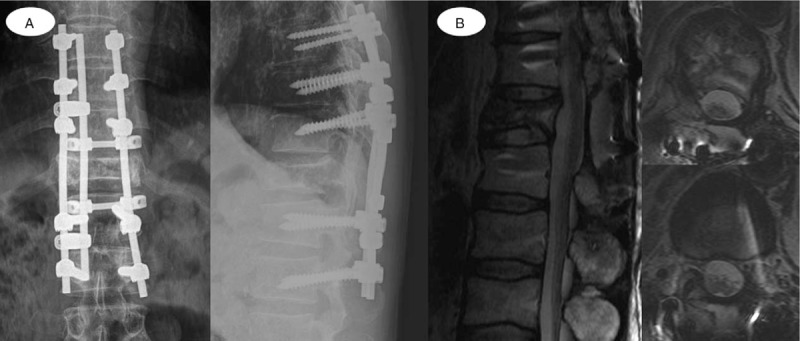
(A) One year postoperatively, simple radiographs (anteroposterior and lateral image) revealed that solid fusion was achieved, with no structural abnormality. (B) Spine MRI 1 year postoperatively showed complete decompression of the spinal cord and resolution of the malignant solitary bone plasmacytoma. MRI = magnetic resonance imaging.
